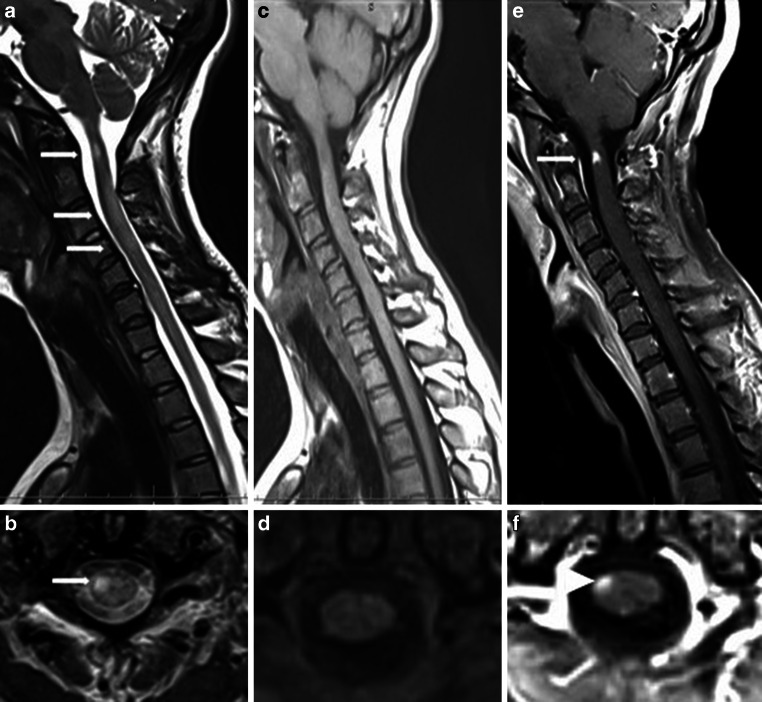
MRI of focal lesions in the spinal cord of a patient with PPMS. a T2-weighted, turbo spin-echo image, sagittal plane. Hyperintense focal lesions at the C1 C3, C4, C5 spinal cord levels (*white arrows*). b Corresponding T2-weighted turbo spin-echo image, axial section at C1 spinal cord level. Hyperintense focal lesions at right side of spinal cord. c T1-weighted, turbo spin-echo, sagittal plane without signal abnormalities and corresponding axial section at C1 spinal cord level (d) also without signal abnormalities. e T1-weighted images after contrast administration, sagittal plane. Gd+ lesion at C1 spinal cord level (*white arrow*) and axial section at the same spinal cord level (f) with Gd+ lesion (*arrowhead*)
